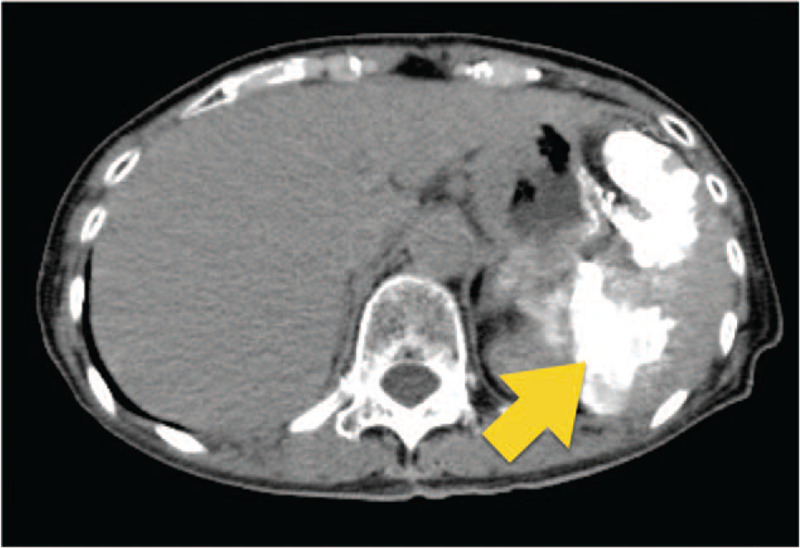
A computed tomography scan with a high absorbed area (arrow) representing an area of splenic embolization. Approximately 70% of the splenic parenchyma is ischemic.
